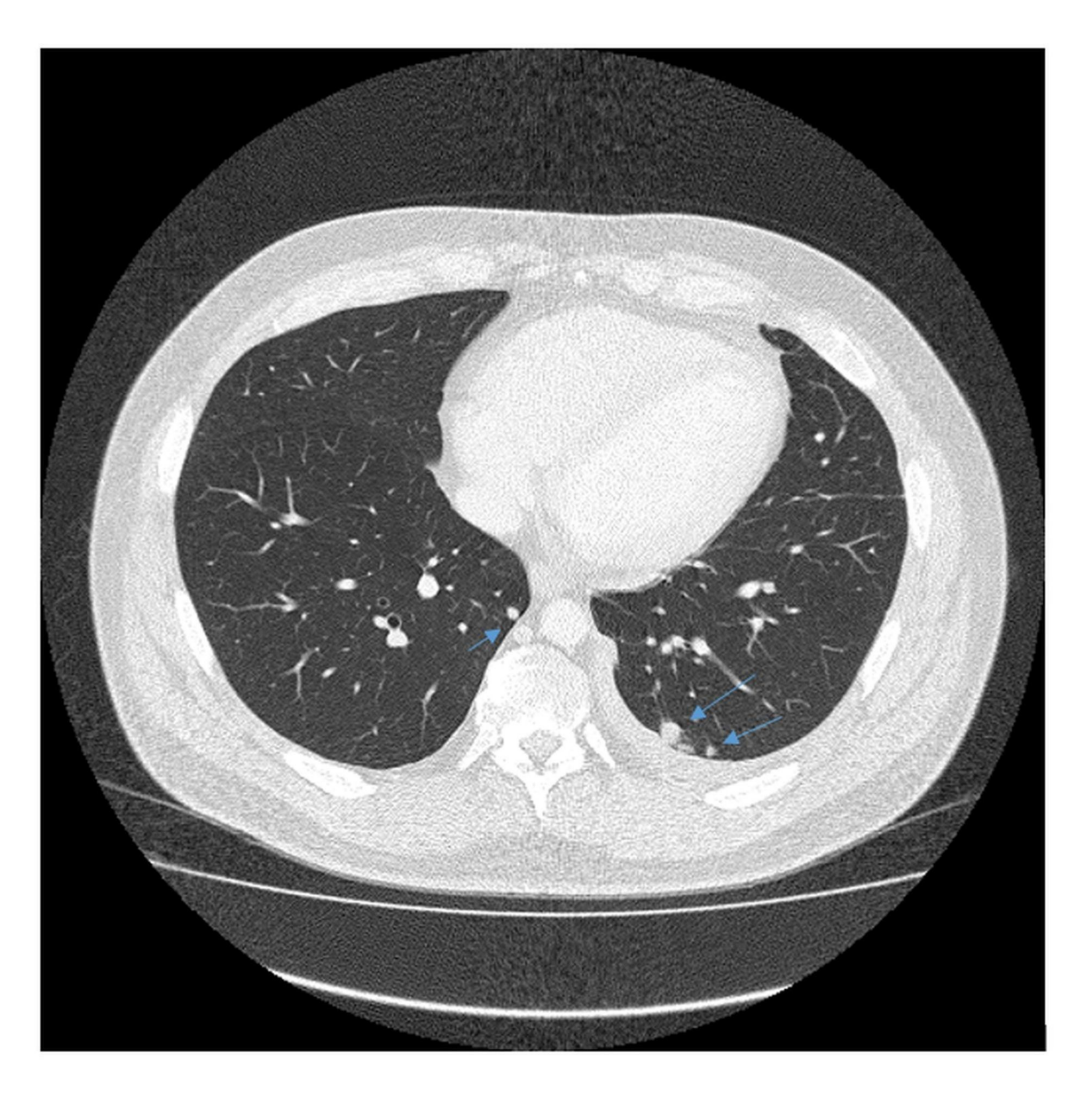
CT scan chest (May 2014) Relapsed Ewing's sarcoma in the lungs with multiple pulmonary nodules CT: Computed tomography
